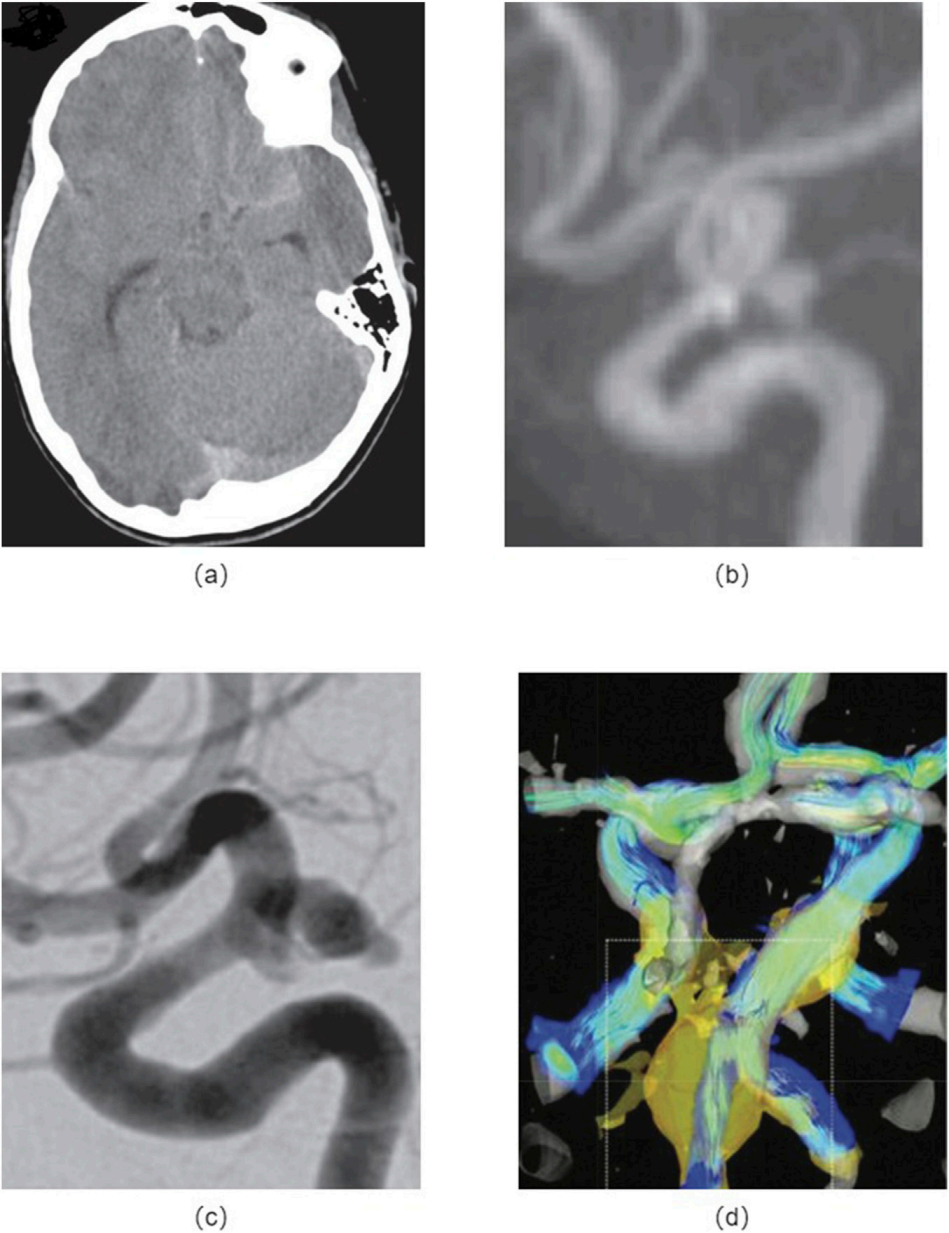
Medical imaging methods: (A) Non-contrast head CT images of a patient with the cerebral aneurysm; (B) MRA shows a left posterior communicating artery aneurysm; (C) DSA indicates an enlarged posterior communicating artery aneurysm; (D) 4D-MRI of the fusiform aneurysm shows the peripheral fast flow channel associated with high WSS.
